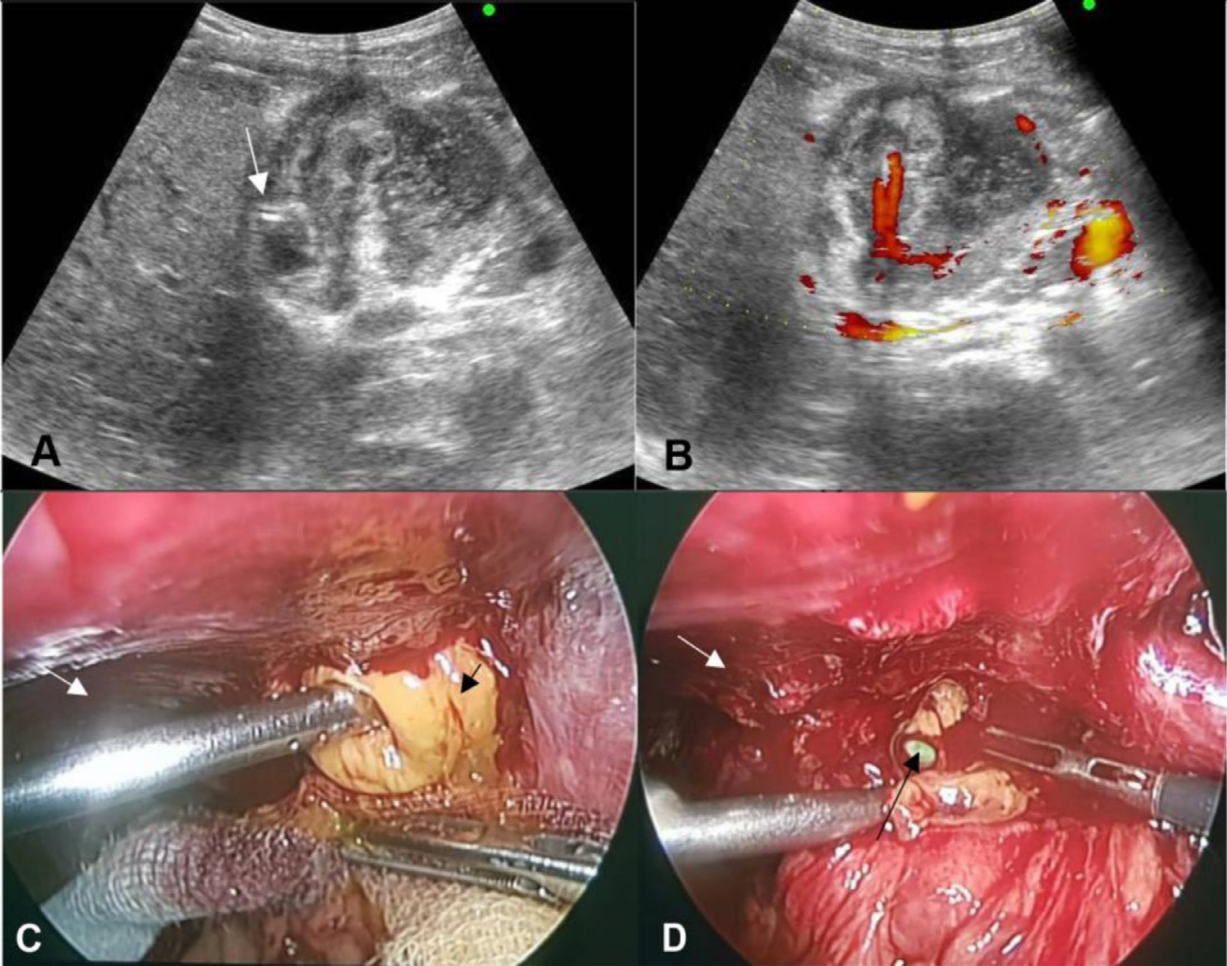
Preoperative ultrasound (US) done on day 50 showed the drain outside the lumen (A), a thickened wall with vascularity on Doppler (B). Laparoscopic view (C) presented a distended dark brown wall mass (white arrow); opening of the wall revealed concentrated yellow purulent fluid (black arrow). After the removal of pus (D) the collapsed gallbladder (white arrow) and catheter drain (black arrow) was seen.
